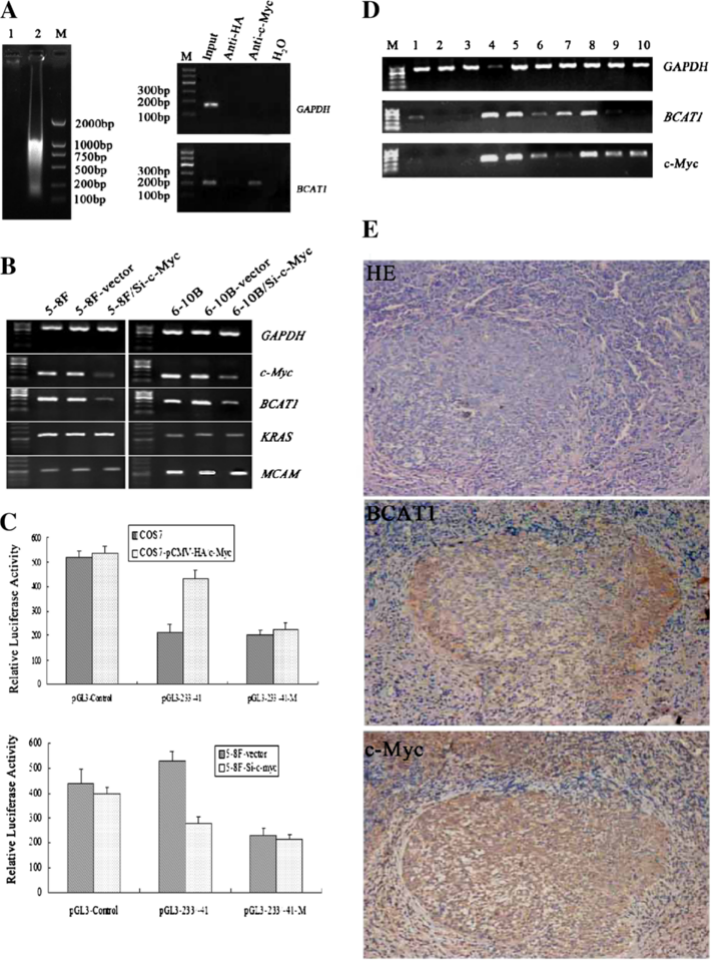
The regulation of *BCAT1 *by c-Myc. (A) ChIP confirmed that transcription factor c-Myc can specifically bind to the regulatory region of *BCAT1*. Lane 1 and 2 represent gDNA untreated or treated with ultrasonication, respectively. (B) Detection of the mRNA level of *BCAT1* in 5-8F cells and 6-10B cells transfected with pRNAT-U6.1/Si-c-Myc vector or blank vector. *BCAT1* mRNA level was reduced when the endogenous expression of *c*-*Myc* was blocked both in 5-8F cells and 6-10B cells, while the expression of *KRAS* or *MCAM*, two non-target genes of c-Myc, was stable despite the change of c-Myc’s level in these cells. (C) Luciferase reporter assay demonstrated the influence of c-Myc on *BCAT1* promoter activity. The results showed that the luciferase activity was positively correlated to the expression level of c-Myc. Here, we used 5-8F-vector cells instead of 5-8F cells as control. (D) The co-expression of *BCAT1* and *c*-*Myc* was detected in NPC tissues by RT-PCR. Lanes 1–3 represent *BCAT1* and *c*-*Myc* expression in CN tissues. Lanes 4–10 represent *BCAT1* and *c*-*Myc* expression in NPC tissues. *GAPDH* was used as an internal control. (E) IHC analysis of the same batch of NPC biopsies demonstrated that BCAT1 and c-Myc were co-expressed in most NPC tissues.
